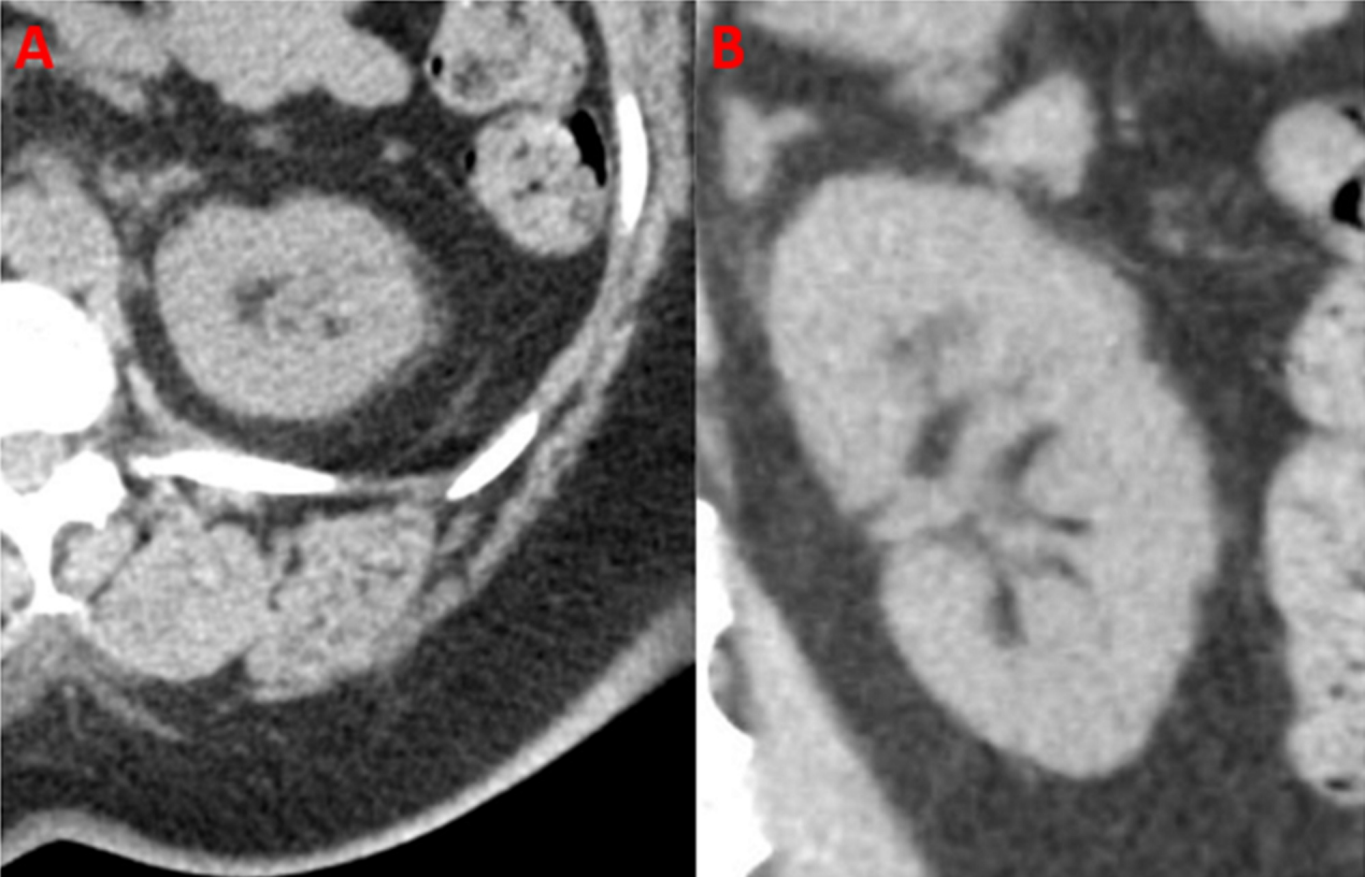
Follow-up computed tomography (CT) after cryoablation. Axial (A) and coronal (B) images show no residual malignancy four months after cryoablation.
